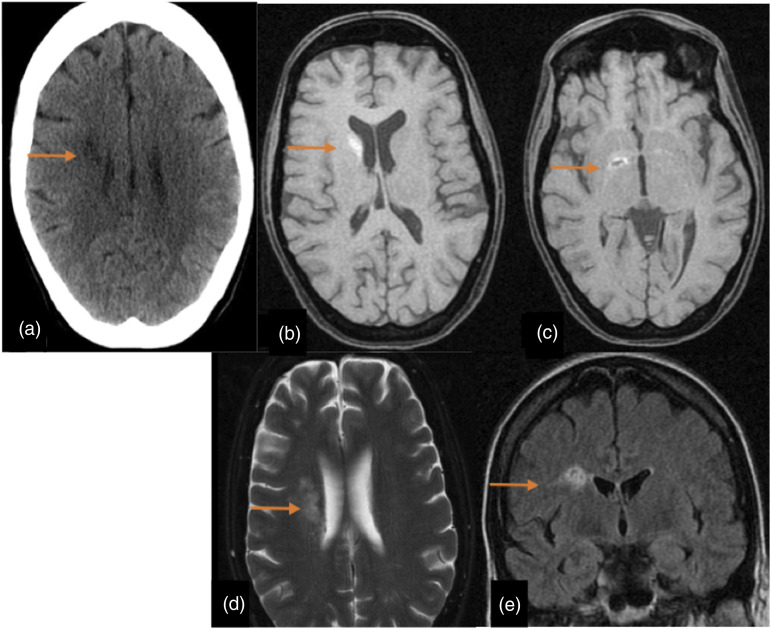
Cryptococcal CNS infection. A 41-year-old woman with primary pulmonary hypertension underwent double-LT. She was diagnosed with disseminated cryptococcal disease and the neuroimaging study was performed to rule out CNS infection. Figures: axial CT scan (a), axial T1-weighted sequences (b and c), axial T2-weighted sequences (d) and coronal FLAIR sequence (e). In CT, there are small hypodense lesions in right corona radiata (arrows).There are three hyperintense small polycystic lesions on the head of the right caudate nucleus, right internal capsule and right corona radiata (arrows).
